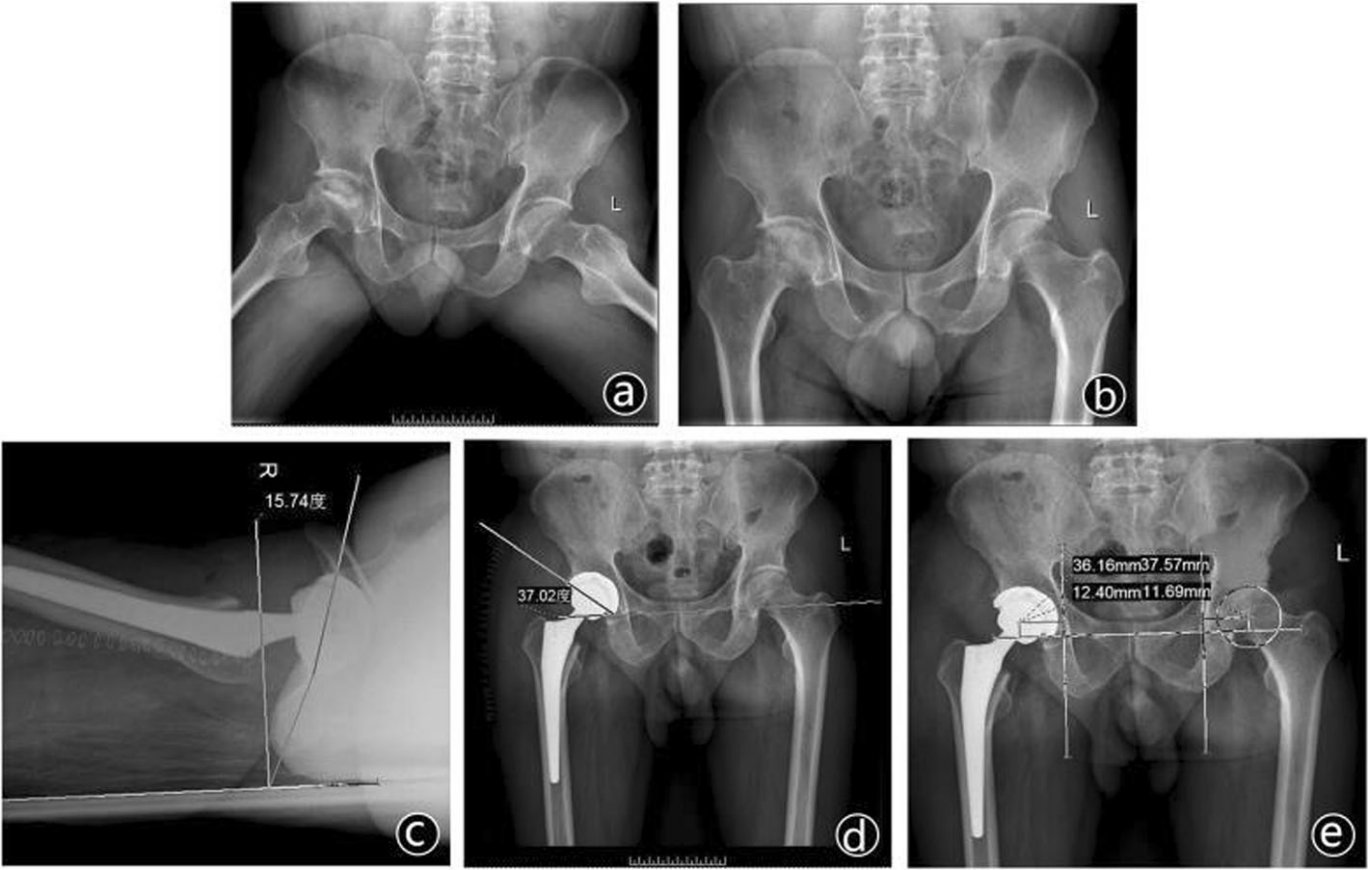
A preoperative radiograph showing osteonecrosis of the right femoral head (a, b). The anteversion angle, inclination angle, and vertical and horizontal displacement on the follow-up radiograph taken 1 year after right THA (c, b, d)
